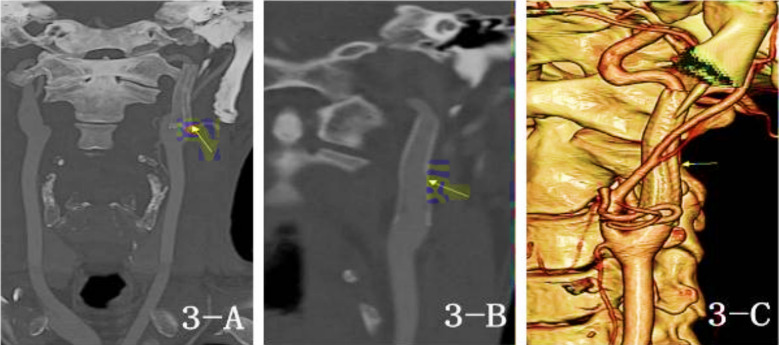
CTA image for 1-year postoperative follow-up Fig.3-A, Fig.3-B: (MIP) and Fig.3-C: (Reconstruction Image) showed the stent had no displacement and the blood flow was unobstructed)
